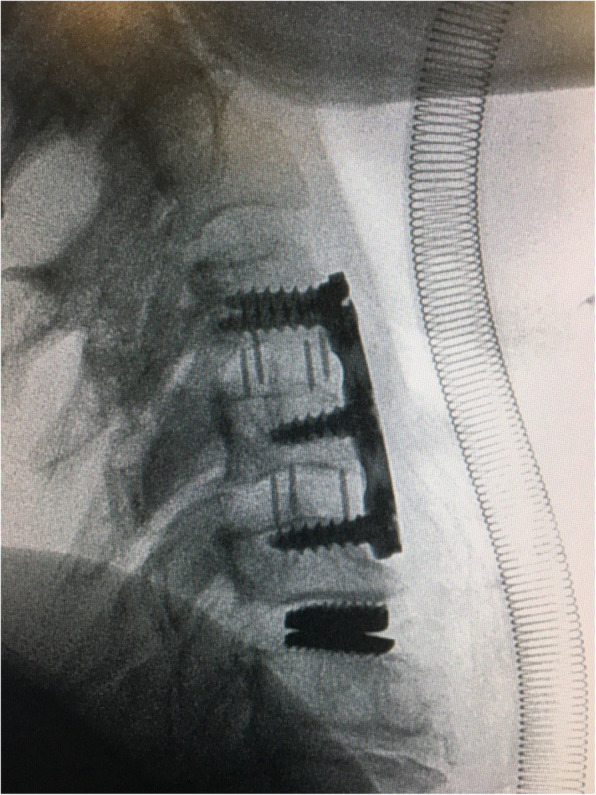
Plain radiograph indicating hybrid construction with anterior cervical fusion for C3–C4 and C4–C5, and cervical disc prosthesis for C5–C6
